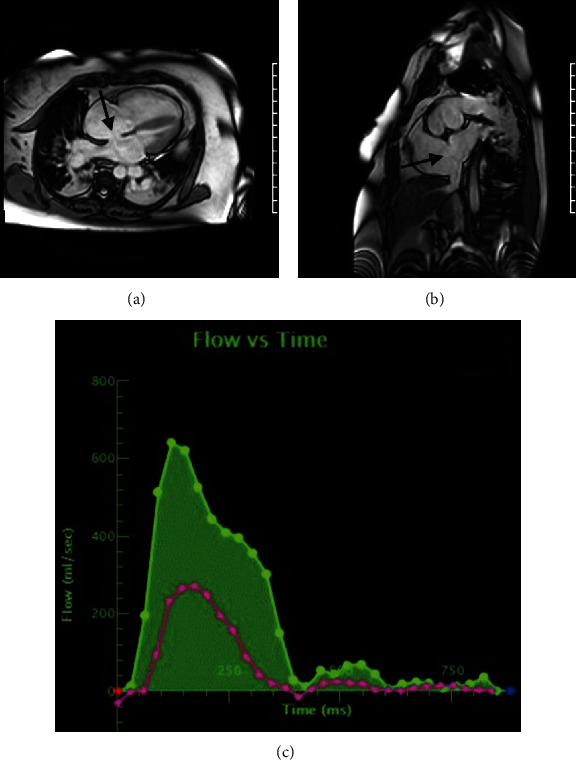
(a) CMR four-chamber view demonstrating dilated right heart with a 21 mm ASD (arrow). (b) CMR short-axis view demonstrating a 21 mm ASD (arrow). (c) CMR QP/QS of 2.9 (pulmonary flow in green and aortic flow in pink). ASD: atrial septal defect; CMR: cardiac magnetic resonance imaging; QP: right ventricular stroke volume; QS: left ventricular stroke volume.
